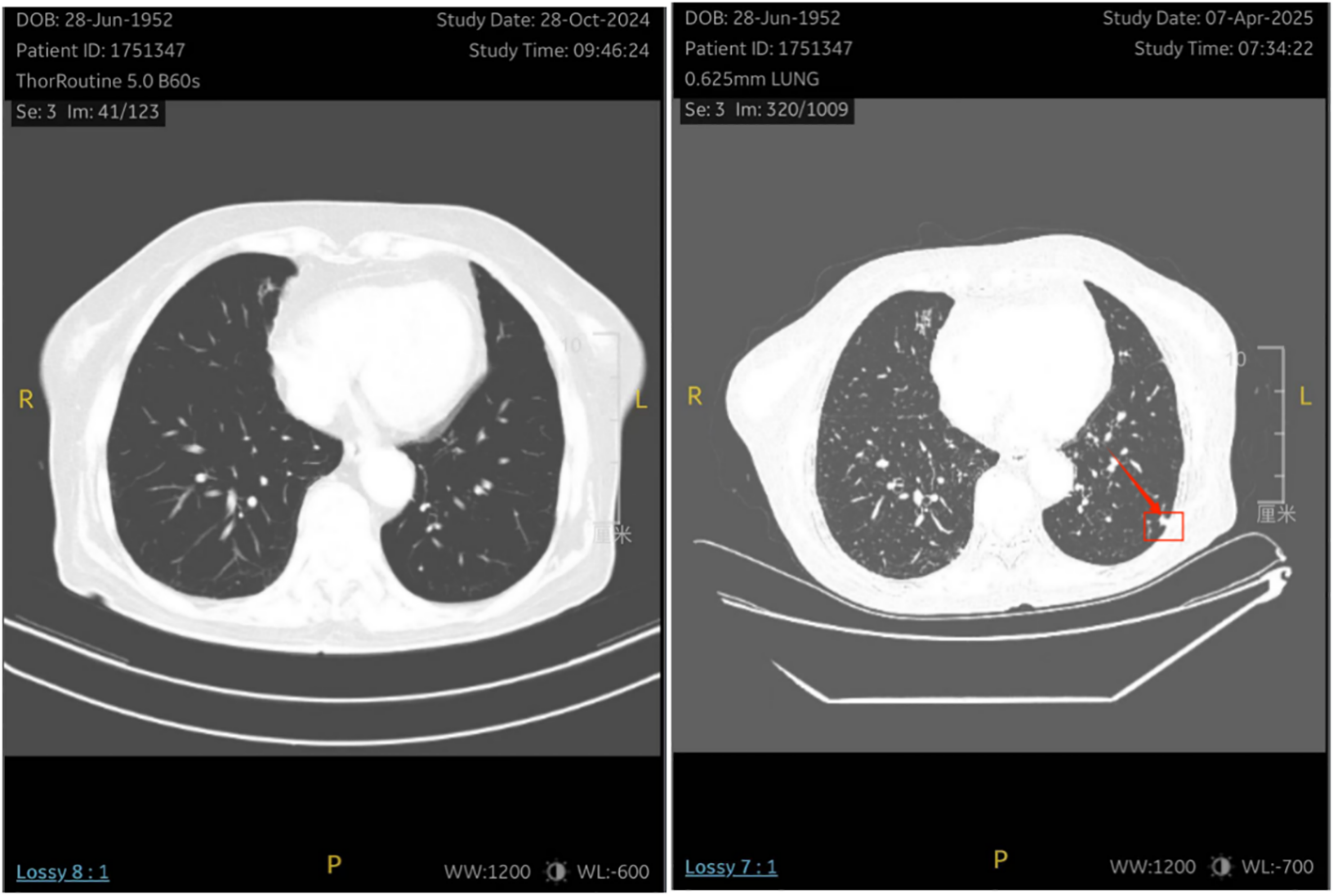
The patient underwent a re-examination of the chest CT on April 7, 2025, which revealed multiple small nodules in the lungs . It is considered that there might be metastatic tumors.
